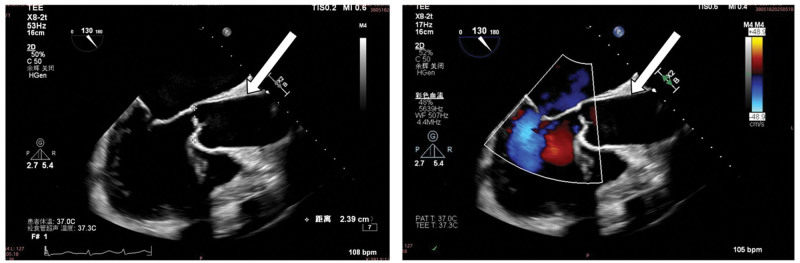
The transesophageal echocardiography shows dilation of the ascending aorta, with no evidence of dissection.
